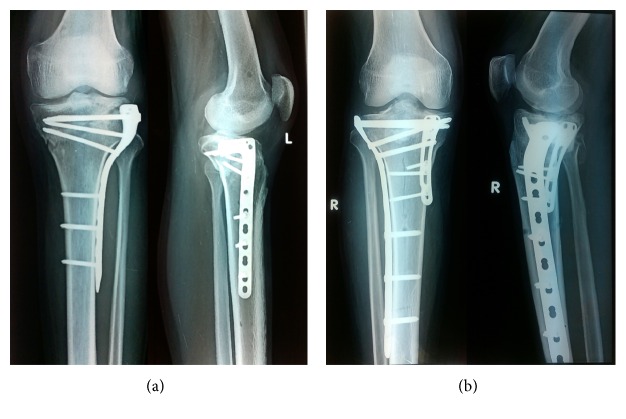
(a) Radiograph showing type V tibial plateau fracture treated with open reduction and internal fixation using lateral type locking plate. (b) Radiograph showing type VI tibial plateau fracture treated with open reduction and internal fixation using medial and lateral locking plates.
